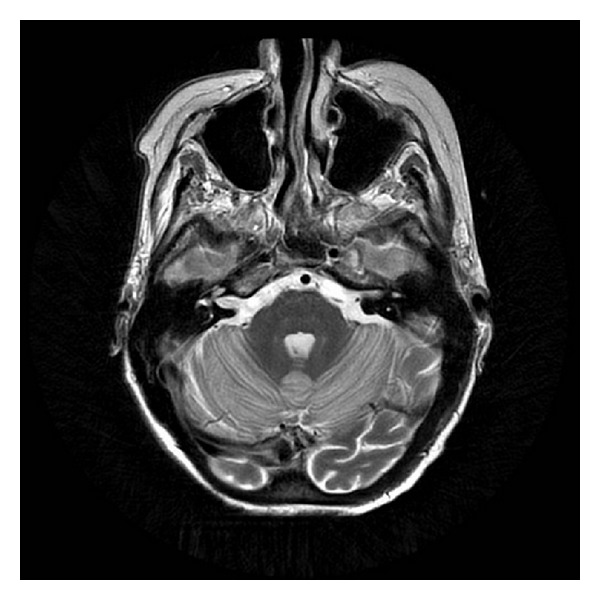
Magnet resonance imaging: axial T2-weighted images showing increased signal intensity in cerebellar hemispheres.
